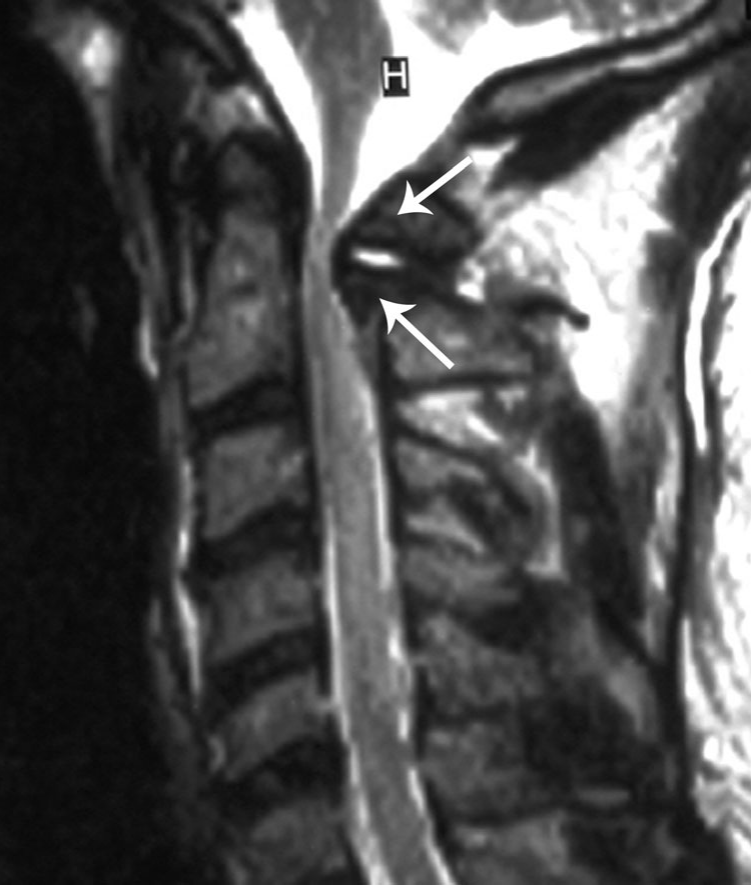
Sagittal T2 weighted MRI demonstrates an expansile lesion (arrows) of the posterior arch of C1 resulting in significant compression on the posterior thecal sac and spinal cord.
